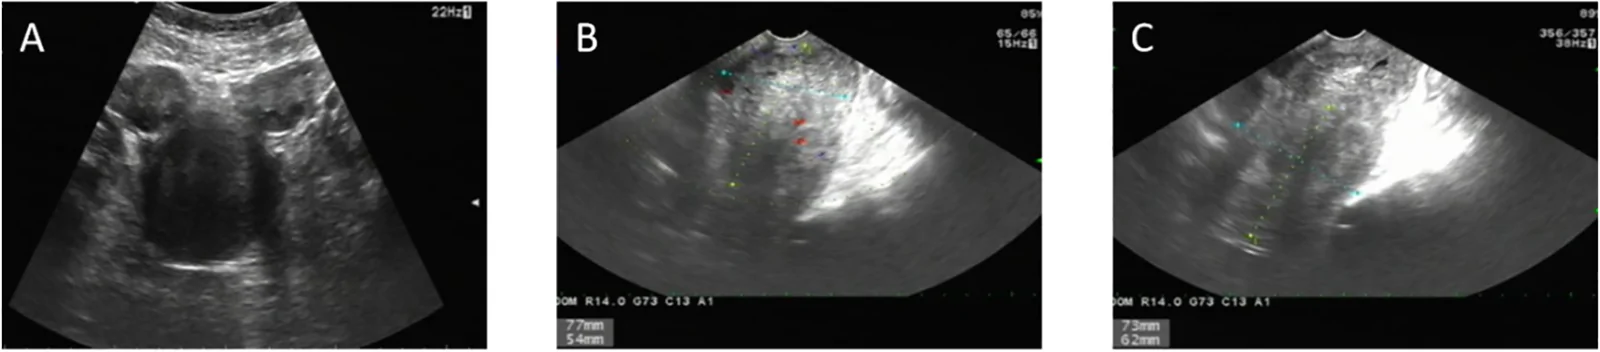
Preoperative gynecological ultrasound of Patient A. (A–C) Transvaginal ultrasound showing a 7.0 × 5.4 cm ill-defined heterogeneous cervical lesion with internal honeycomb cystic changes and hypervascularity on CDFI.
